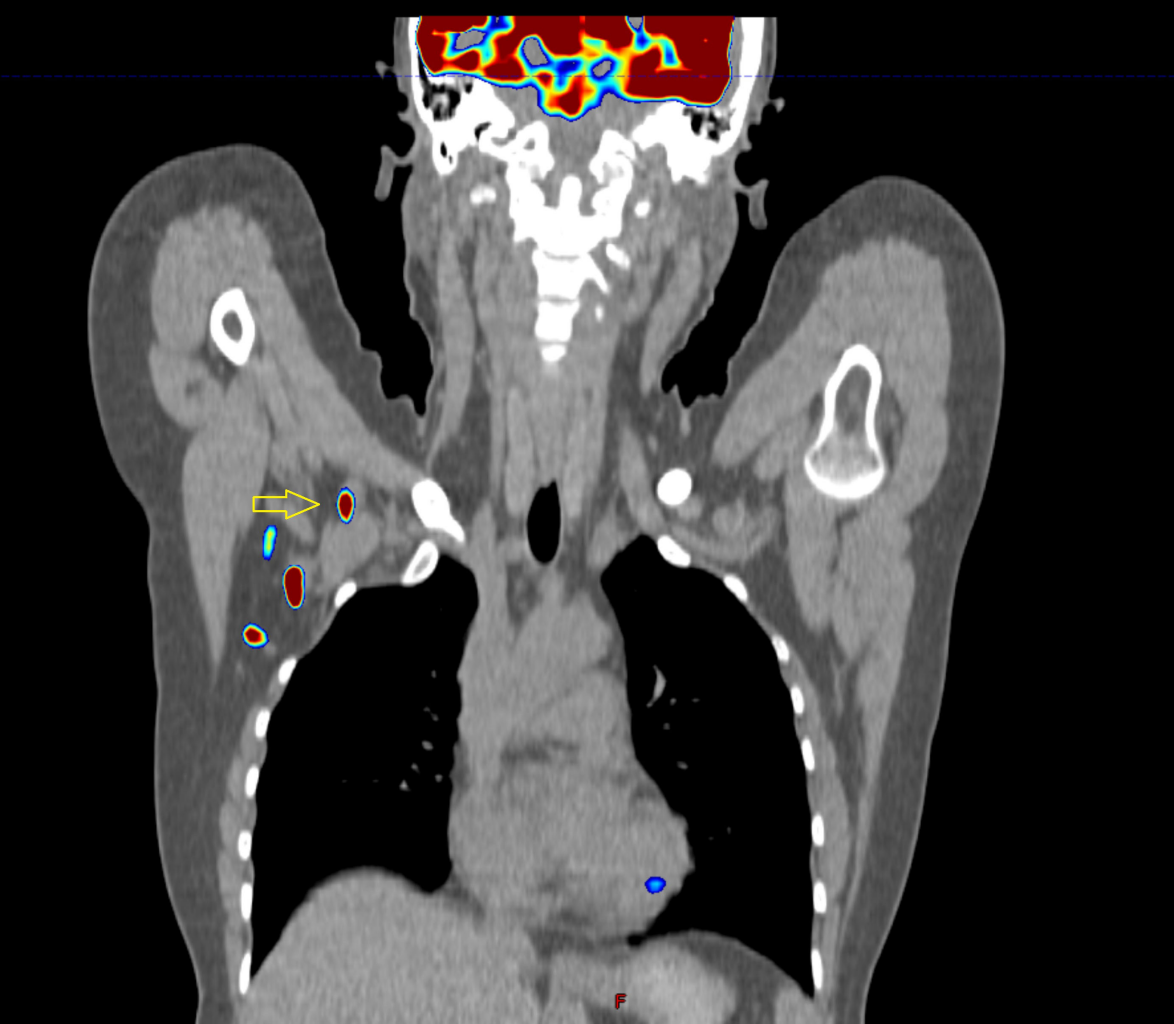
Images of a patient after axillary lymph node dissection, two out of eleven nodes contained metastases. Is this lymph node (yellow arrow) visible on baseline PET-CT operable? What has happened to the third node? It is very likely that it remained in the axilla, despite the surgeon’s efforts.
